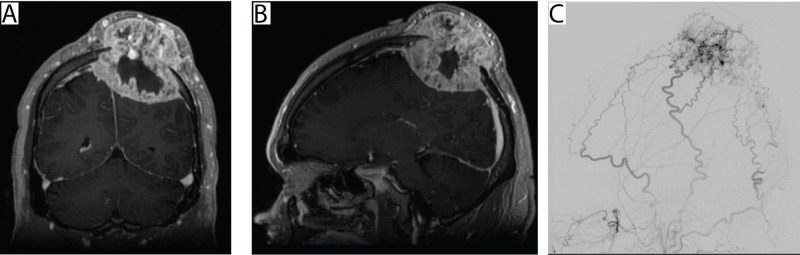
Preoperative imaging. A: Postcontrast T1 weighted coronal magnetic resonance (MR) image showing a calvarial-based metastatic lesion invading the calvarium over the left parietal-occipital region. B: Postcontrast T1 weighted sagittal MR image showing the same lesion. C: Preoperative angiogram showed that the tumor had arterial supply from multiple branches of the bilateral superficial temporal, middle meningeal, and occipital arteries as well as intracranially from the left anterior falcine artery.
